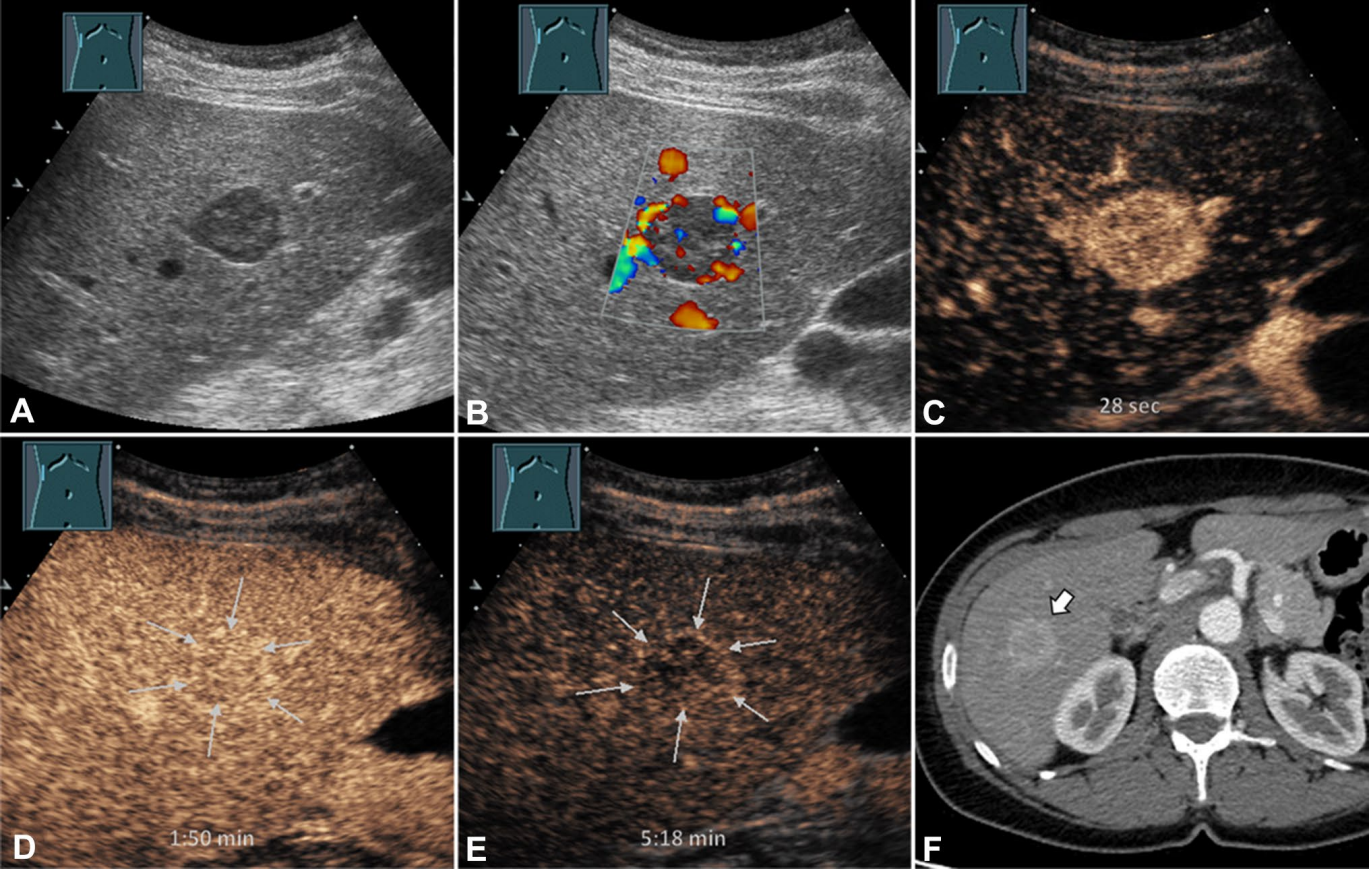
(A) B-mode ultrasound of a 51-year-old asymptomatic patient with hepatic PEComa shows a hypoechoic lesion in segment V of an otherwise unremarkable liver. (B) On color Doppler ultrasound peripheral flow signals were seen. Contrast-enhanced ultrasound of the liver in a patient with proven PEComa (C–E). The lesion shows a marked homogeneous enhancement 28 s after administration of SonoVue® (C). In the portal phase, an isoenhancement of the lesion (arrows) persists (D). In the late phase, mild central washout with a persistent rim enhancement of the lesion (arrows) is recognizable (E). Computed tomography (courtesy of Professor Dr Mahnken, Department of Radiology, University Hospital Marburg) shows a hepatic PEComa with strongly accentuated rim in the arterial phase (F)
